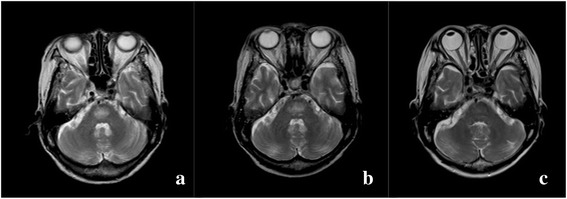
Brain magnetic resonance scan. The hyperintense lesion in the central pons in T2-weighted magnetic resonance imaging decreased and was almost undetectable after several courses of chemotherapy. (a) Before treatment, (b) after two courses, and (c) after seven courses of chemotherapy
